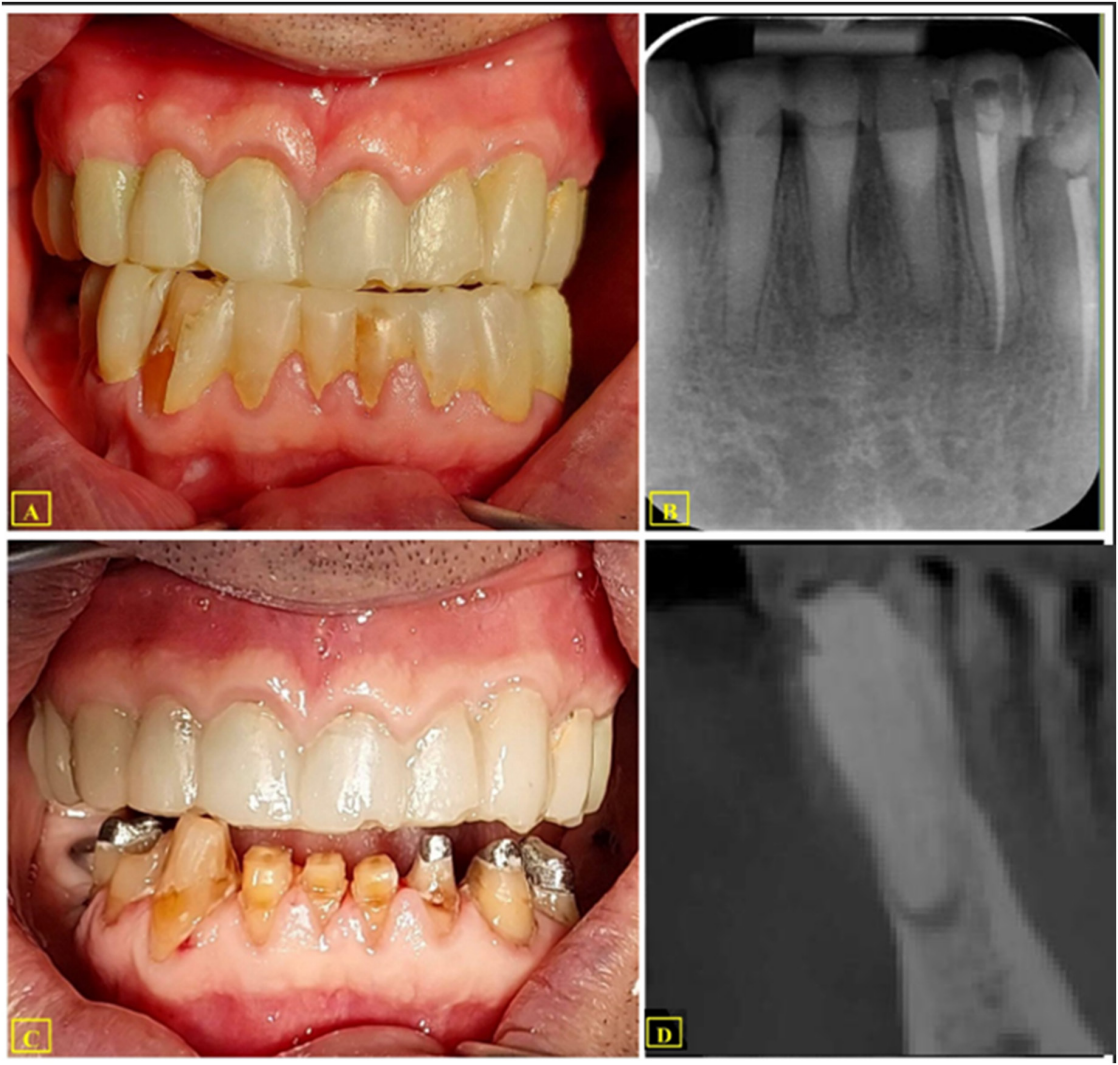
(A) the clinical view; (B) The periapical radiograph (it was 2 months before the start of the root canal treatment); (C) the mock‐up removal and incisal reduction of the teeth; (D) CBCT, Sagital view of tooth #41.
